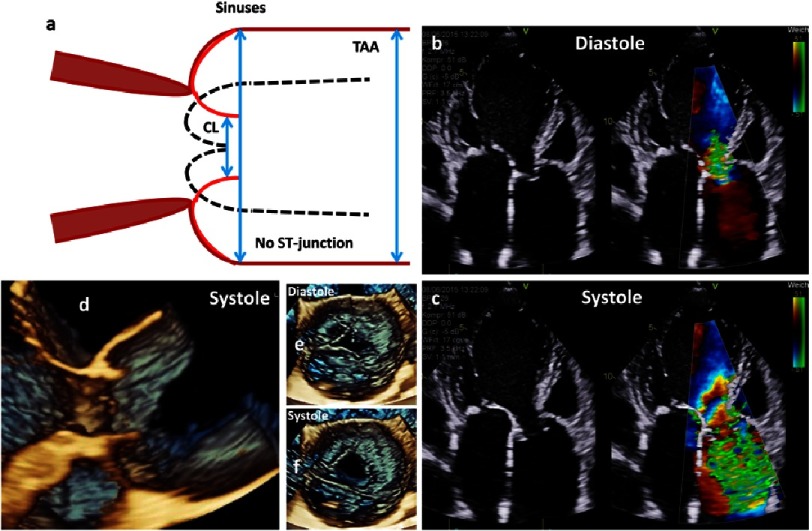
The scheme shows an aneurysm of the sinuses of Valsalva with an ectasia of the proximal ascending aorta and disappearance of the ST-junction and the direct transition of the sinuses into the proximal TAA (a). Further, native and color-coded 2D transthoracic images during diastole (b) and systole (c), 3D transthoracic long axis view during systole (d) and 3D transesophageal en-face views of the AV during diastole (e) and systole (f) are shown.
